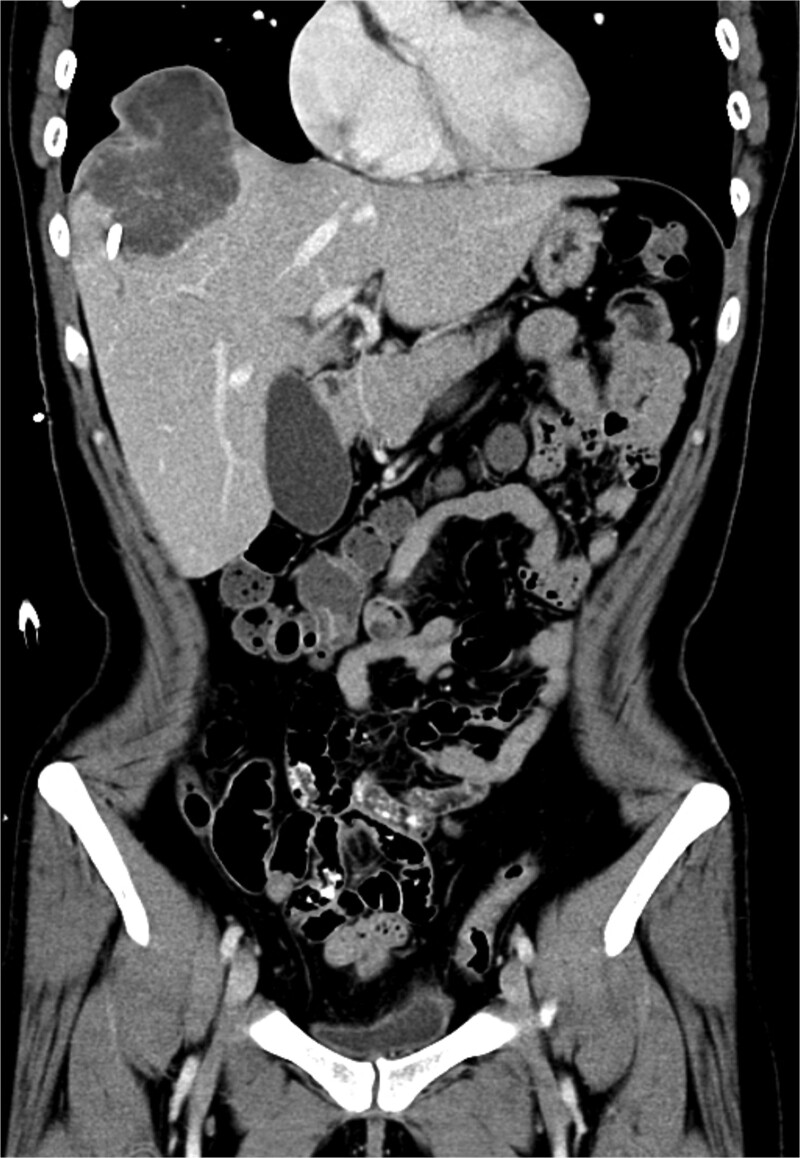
Coronal view of a CT image of sarcomatoid cholangiocarcinoma.
